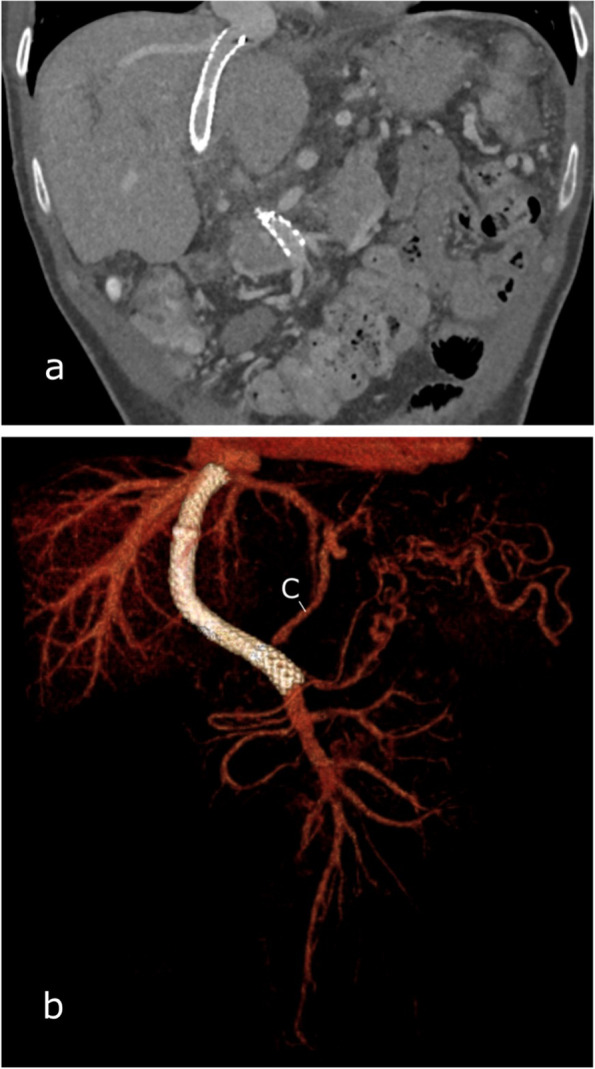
Post-interventional computed tomography (CT) on the day after TIPS insertion. The TIPS is patent, and the portosystemic collaterals have decreased in size (C). a Coronal view with maximum intensity projection (5 mm). b Coronal view with 3D volume rendering technique reconstruction
